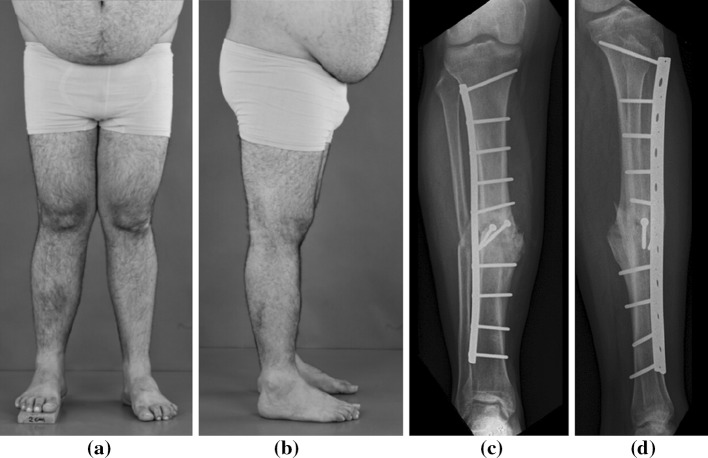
Visualization of surgical result (10 months postoperatively). a Frontal and b lateral photographic images. c AP and d lateral radiographs
